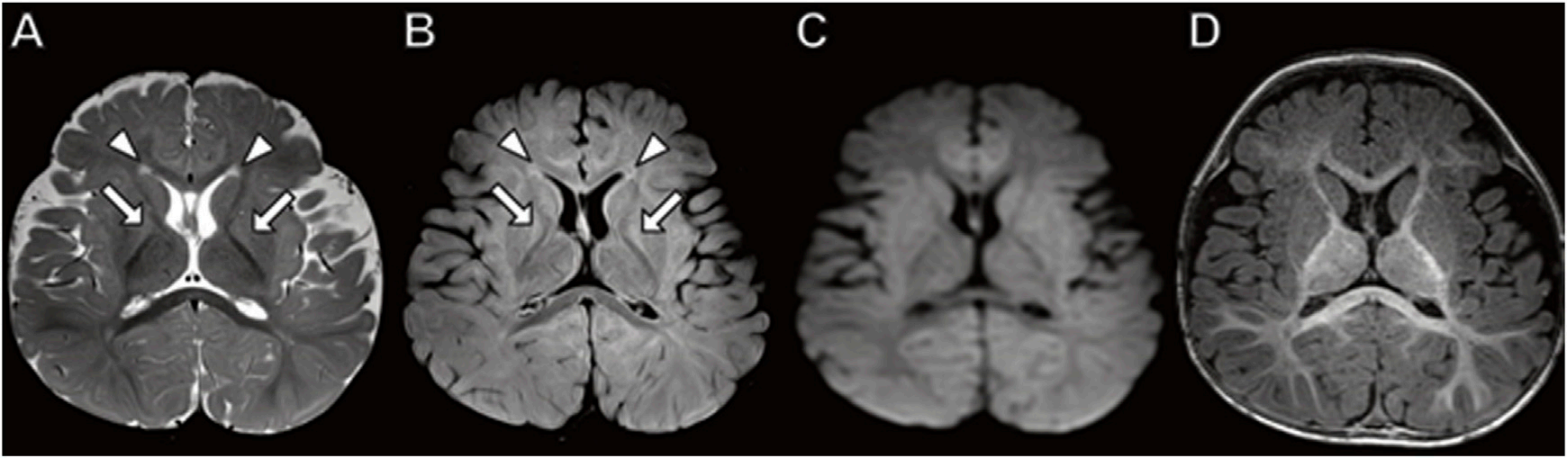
Brain MRI in 7-month-old boy with *MICU1* mutation. (A) Axial T2 and (B) axial fluid-attenuated inversion recovery (FLAIR) images demonstrate a mild signal hyperintensity in the globus pallidus (arrows) and frontal horn periventricular white matter (arrowheads). No abnormal signal on diffusion-weighted (C) or T1-weighted (D) images.
